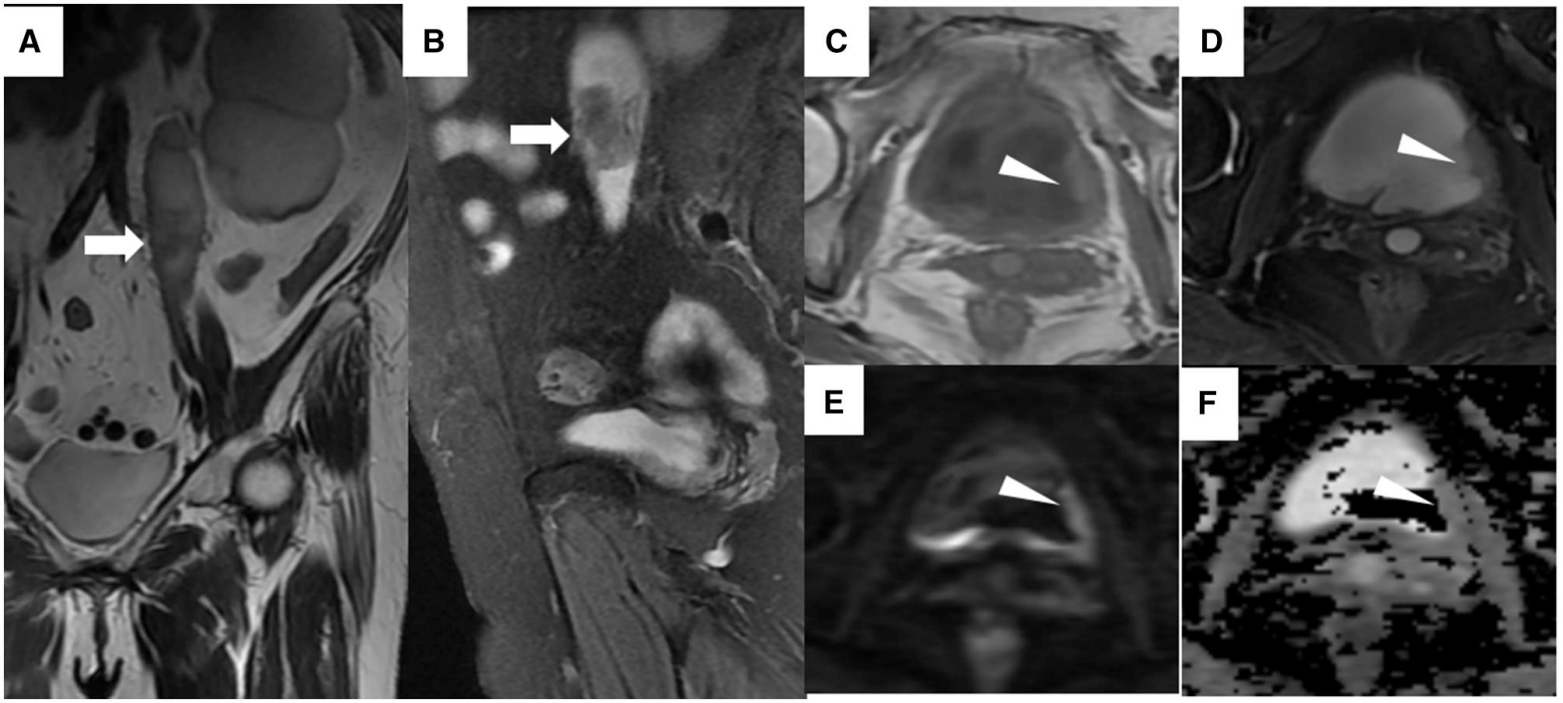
Magnetic resonance imaging of ureteral and bladder malakoplakia. (A) Coronal T2-weighted and (B) sagittal fat-saturated T2-weighted images demonstrate a hypointense intraluminal mass (arrow) in the left proximal ureter. (C) Axial T1-weighted and (D) axial fat-saturated T2-weighted images reveal asymmetric thickening of the left posterior bladder wall (arrowhead). (E) Axial diffusion-weighted image and (F) the corresponding apparent diffusion coefficient (ADC) map show mild restricted diffusion within the thickened wall.
